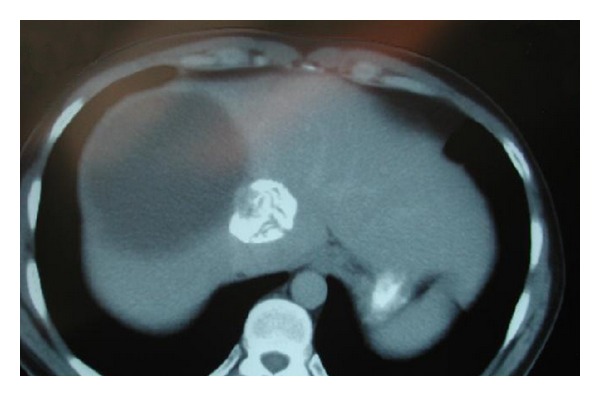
CT after 3 month course of albendazole and praziquantel.
